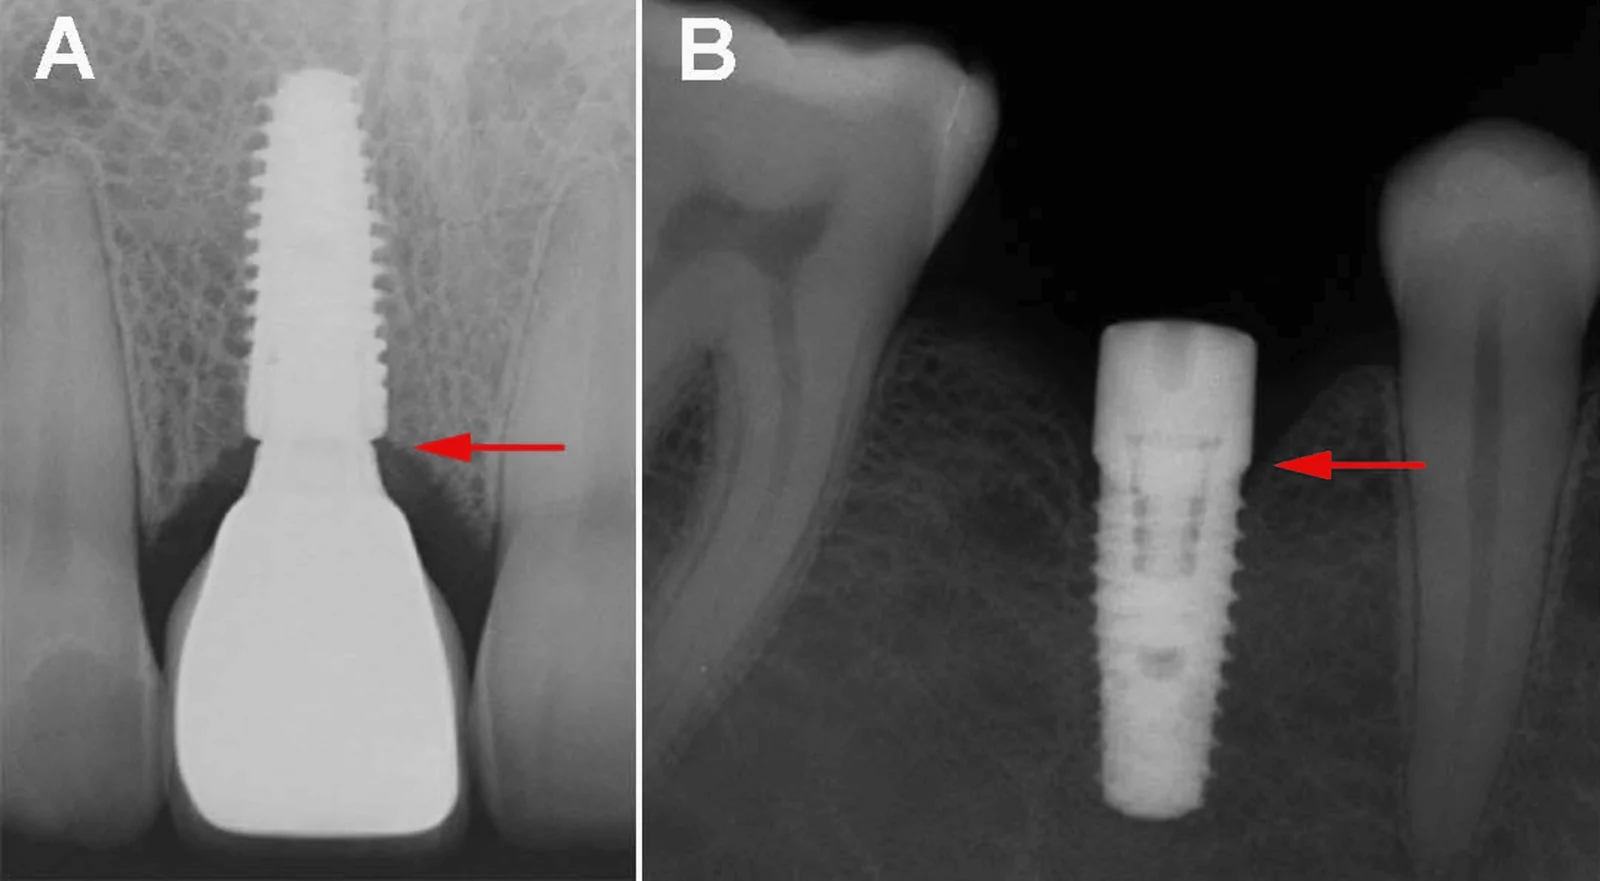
Intraoral periapical radiographs showing abutment type (red arrows) (A) Platform switch-type abutment in the maxillary central incisor region; (B) Platform match-type abutment in the mandibular first molar region. Images acquired from original patient data.
